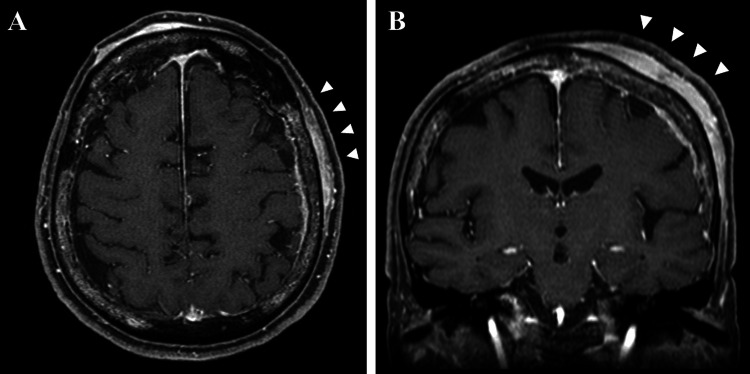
Contrast-enhanced MRI after the first course of multiagent chemotherapy Marked tumor shrinkage was observed (arrowhead, axial (A), and coronal view (B)).
